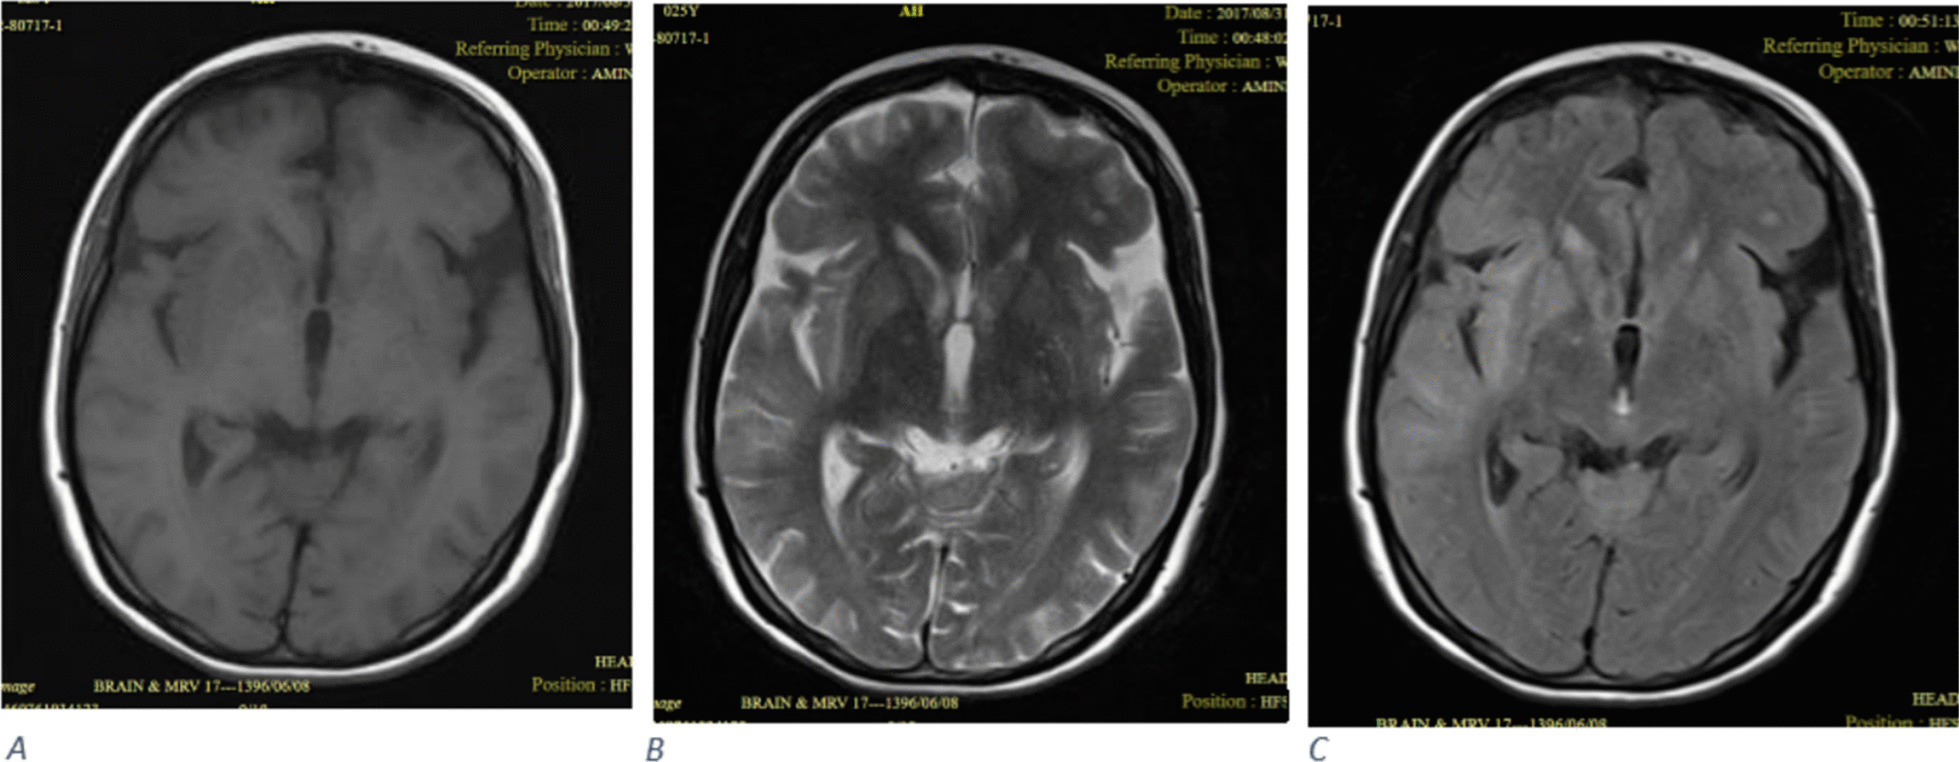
A T1-weighted image shows low signal intensity involving right frontotemporal lobe. B T2-weighted image demonstrated increased signal intensity in the right frontotemporal lobe. C FLAIR image demonstrated increased signal intensity in the right frontotemporal lobe
